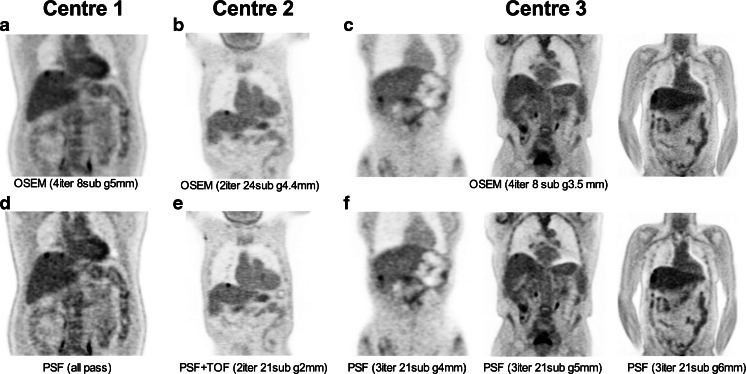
Representative images of OSEM (a, b, c) and PSF or PSF+TOF (d, e, f) reconstructions for the three participating centres. All images have been scaled on the same maximum value (SUV = 5). All selected patients are patients with liver metastases from colorectal cancer. Note the increase in apparent tracer uptake in PSF or PSF+TOF images as compared to a conventional OSEM algorithm fulfilling the EANM requirements. This increase was particularly present in the PSF or PSF+TOF images without filter or when applying a Gaussian filter with a small kernel (centres 1 and 2)
